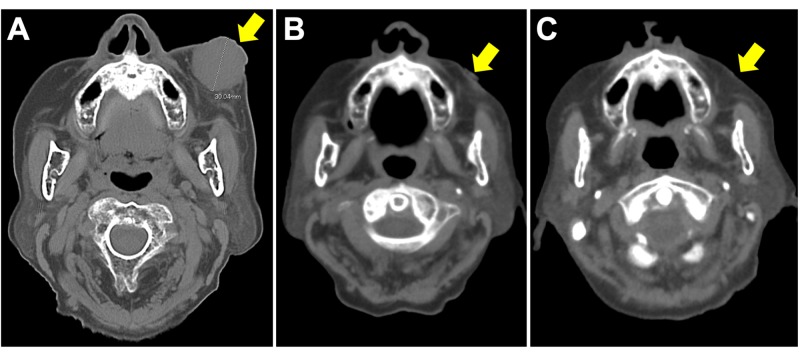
Computed tomography images of a Merkel cell carcinoma of the left cheek (A) Before initiation of radiation therapy; (B) at the completion of radiation therapy; (C) three months after initiation of radiation therapy; arrows indicate the tumor site.
